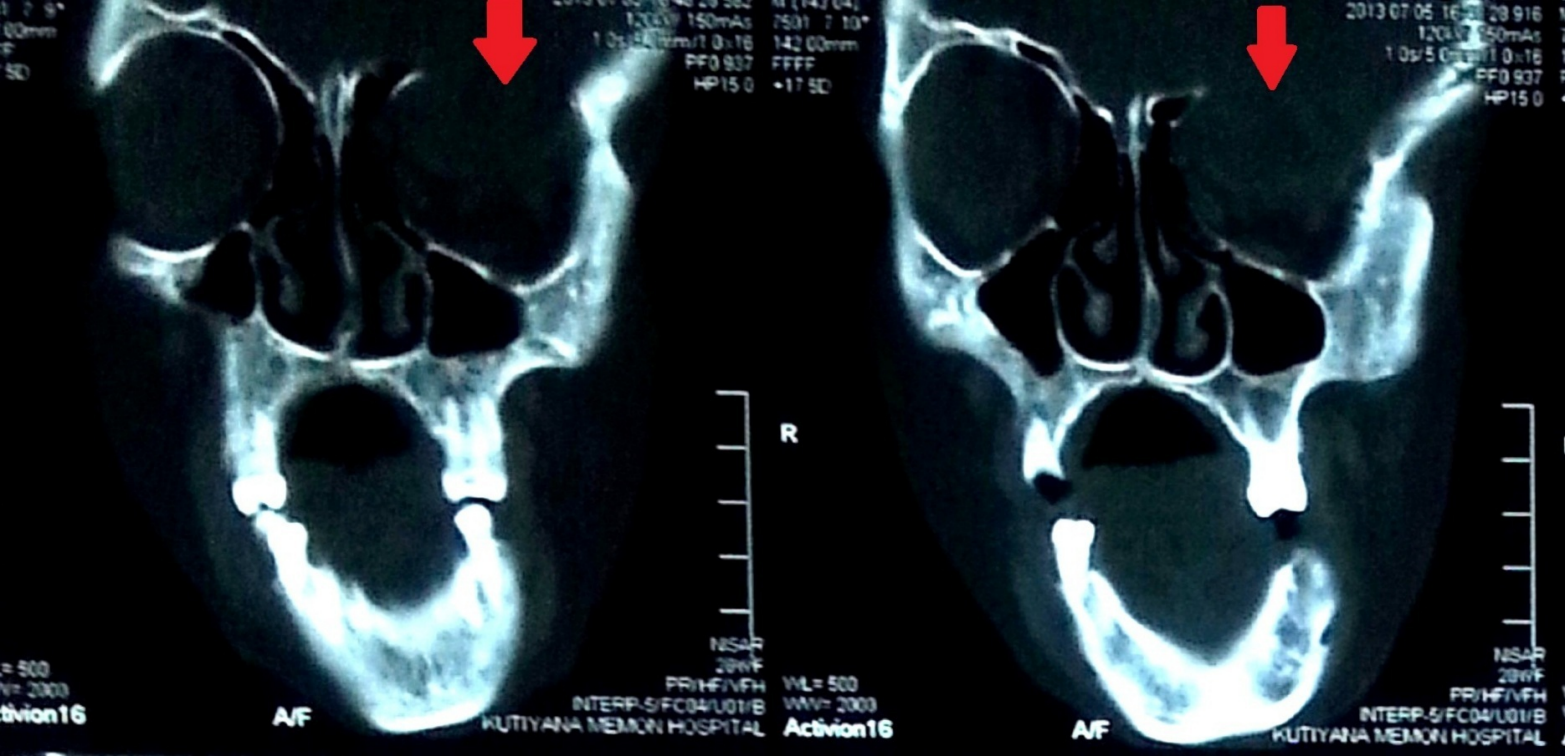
CT scan bone window coronal views showing deficient roof of the left orbit. CT: Computed tomography.
